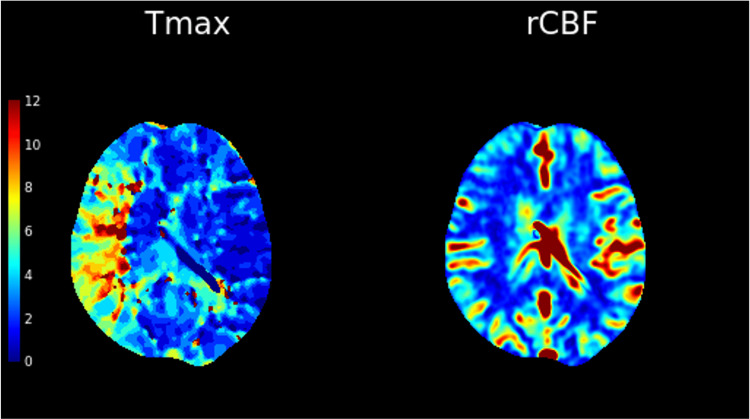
CT perfusion maps done as a part of the stroke protocol show a large area of perfusion mismatch between the time-to-maximum (Tmax) and the relative cerebral blood flow (rCBF) in the right middle cerebral artery territory. This signifies salvageable ischemic brain tissue that will benefit from endovascular treatment.
